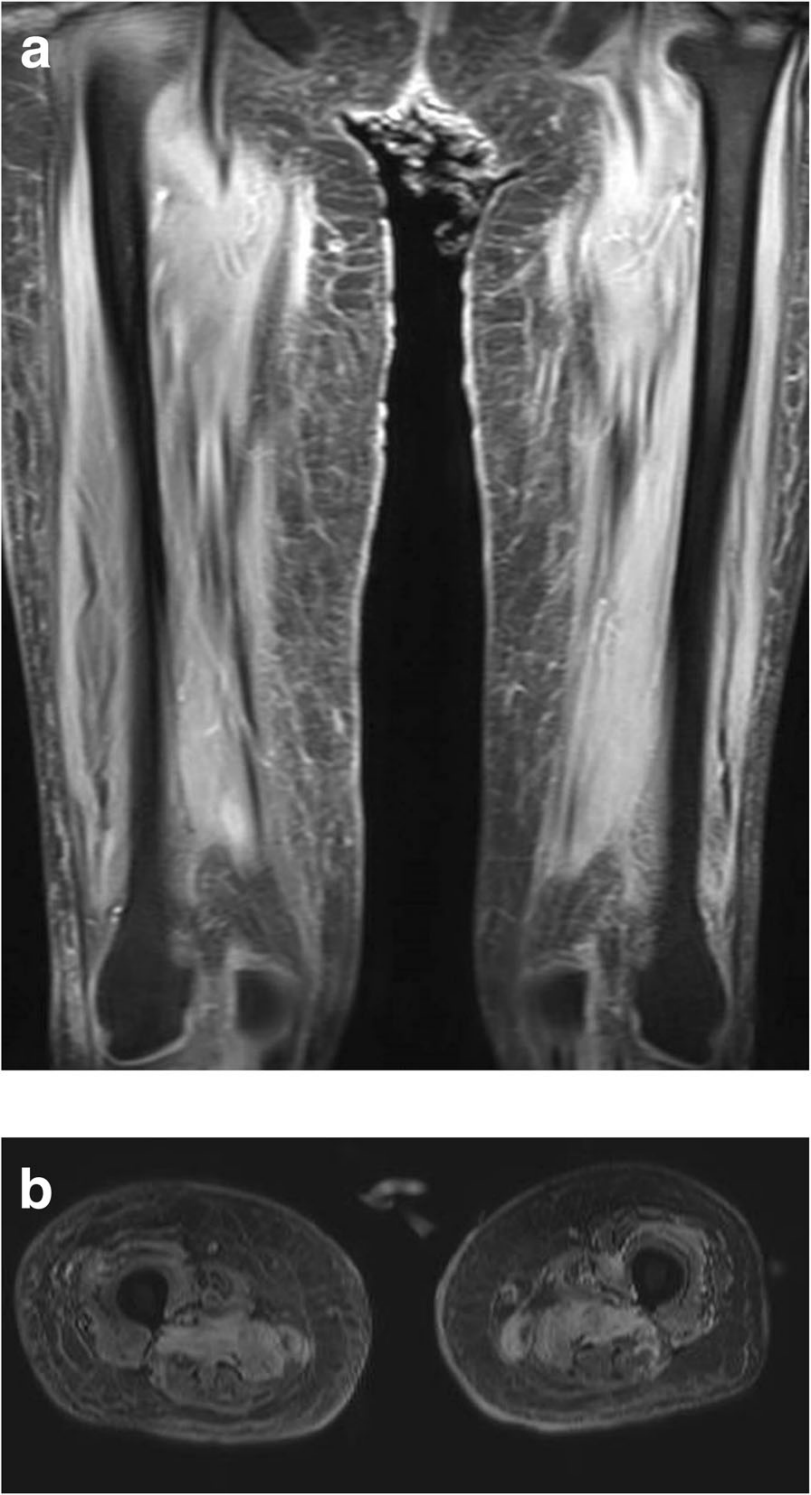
a. Coronal T2-weighted MRI of the thighs. b. Axial T2-weighted MRI of the thighs demonstrating enhancement of subcutaneous tissue and muscle. MRI, magnetic resonance imaging
